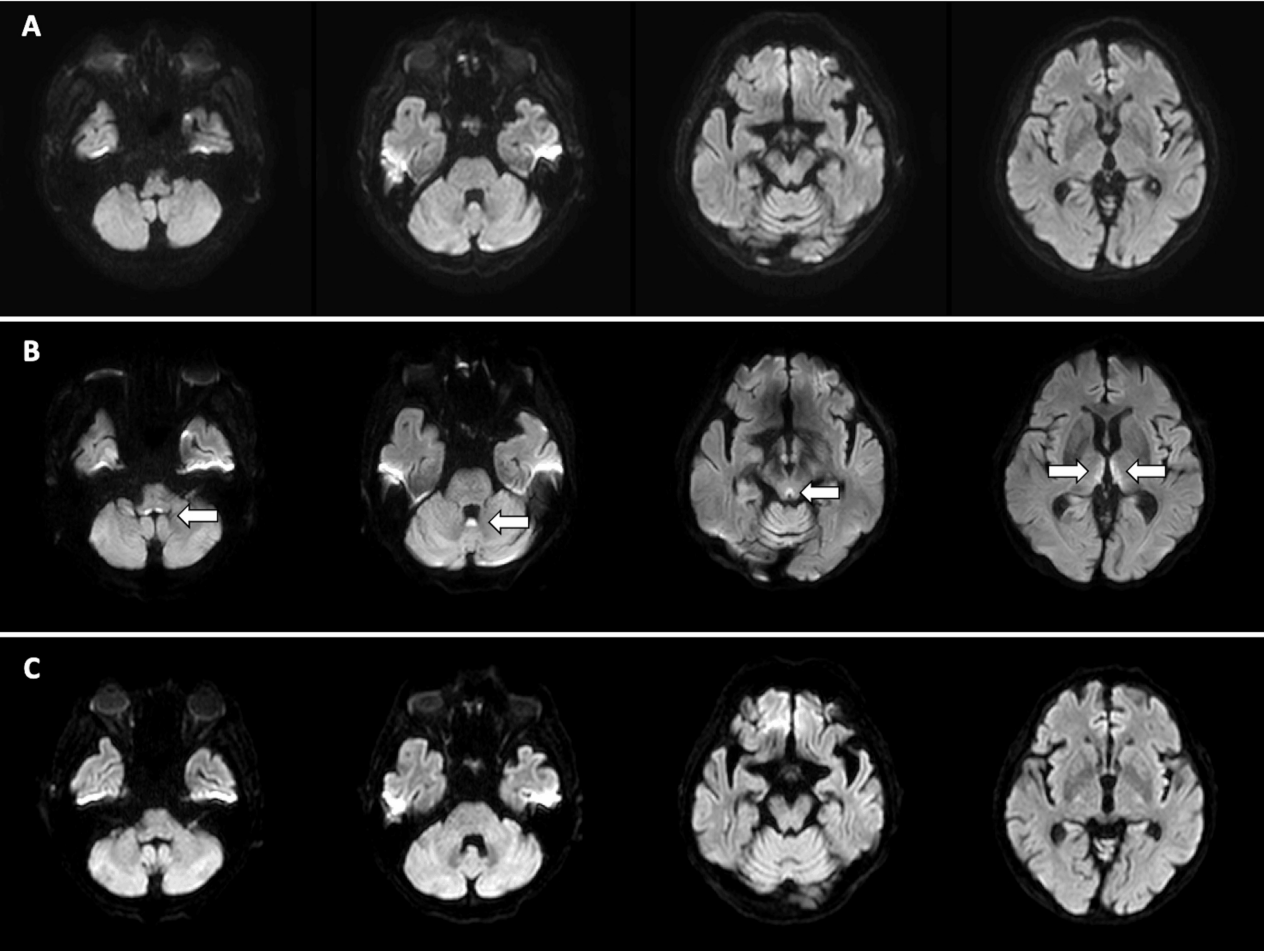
DWI findings over time. (A) DWI on admission revealed no abnormalities. (B) DWI on day 4 revealed hyperintense lesions in the bilateral dorsomedial thalamus, mammillary bodies, periaqueductal gray matter, dorsal medulla, and cerebellar vermis (arrows). (C) DWI showed that all abnormalities had disappeared by day 17. DWI, diffusion-weighted imaging
